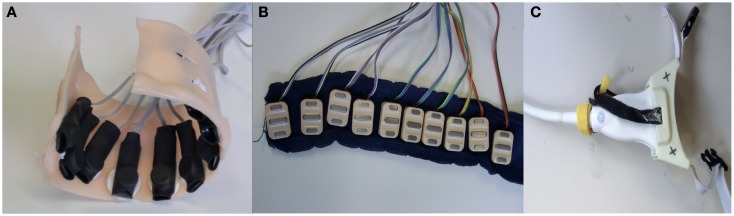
HMI devices used: (A) customized arrangement of FSR housed in a semi-rigid bracelet; (B) ten sEMG electrodes arranged on a strip of bio-compatible self-adhesive tape; (C) ultrasound transducer fixed to a custom-made cradle.
